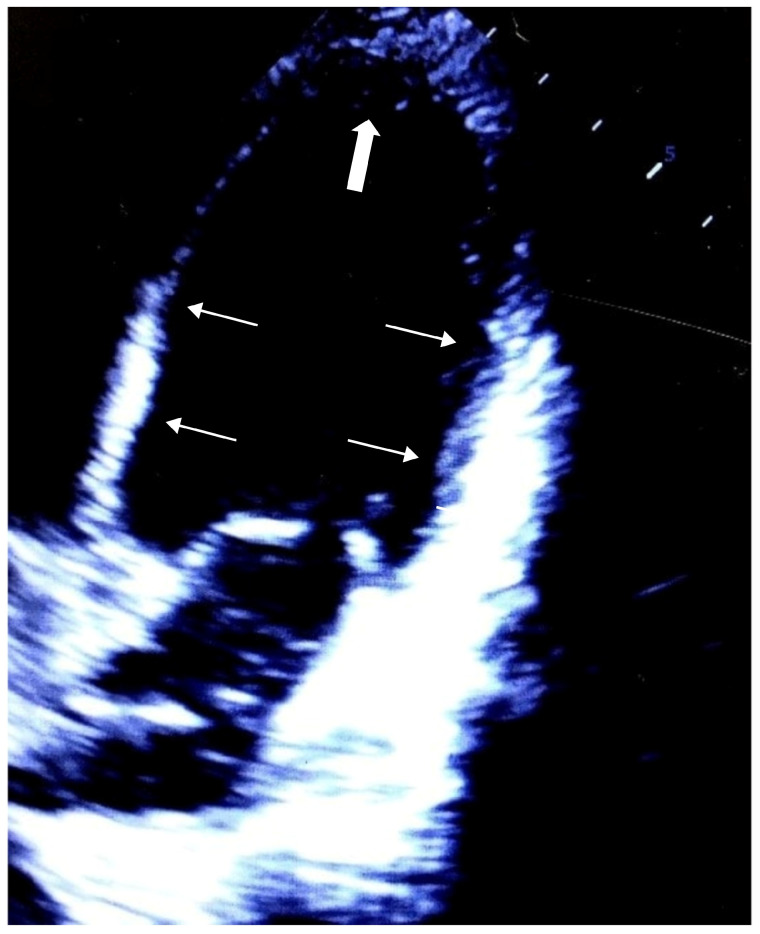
Echocardiography showing a severe compromise of cardiac contractility with regional wall motion abnormalities (thin arrows), apical hyperkinesia (thick arrow), along with reduced left ventricular ejection fraction (EF) of 40%, and fractional shortening (FS) of 28%. At end-systole the left ventricle resembles the basal ballooning pattern, giving the appearance of reverse Takotsubo cardiomyopathy (Video Recording of the Echocardiography: https://doi.org/10.6084/m9.figshare.20526903).
